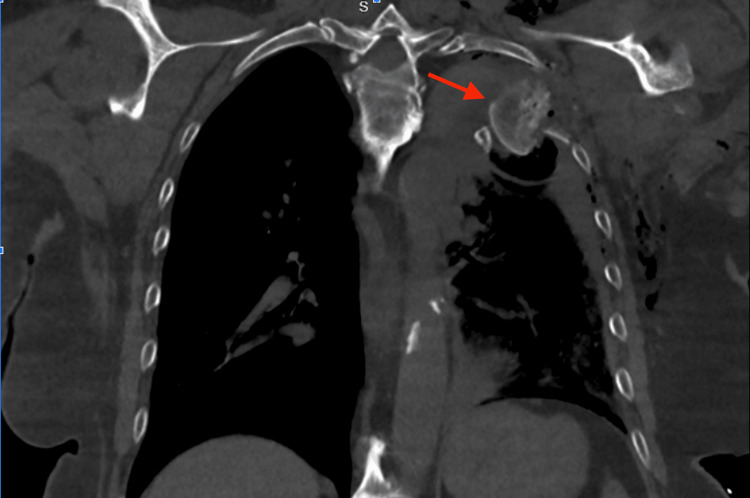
CT in coronal view showing the comminuted left humeral fracture with fragments of the humeral head penetrating through the left chest wall and pleura (red arrow)
